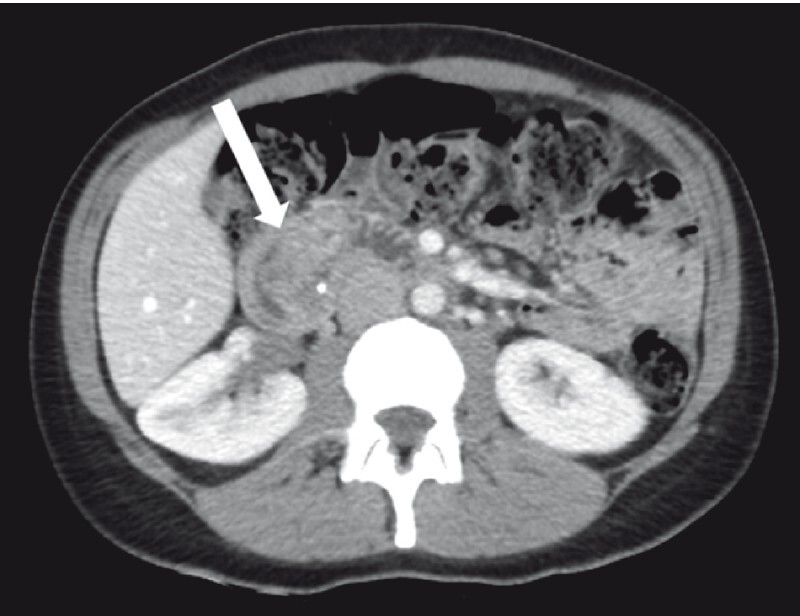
Computed tomography image showing the large duodenal polyp (arrow).
